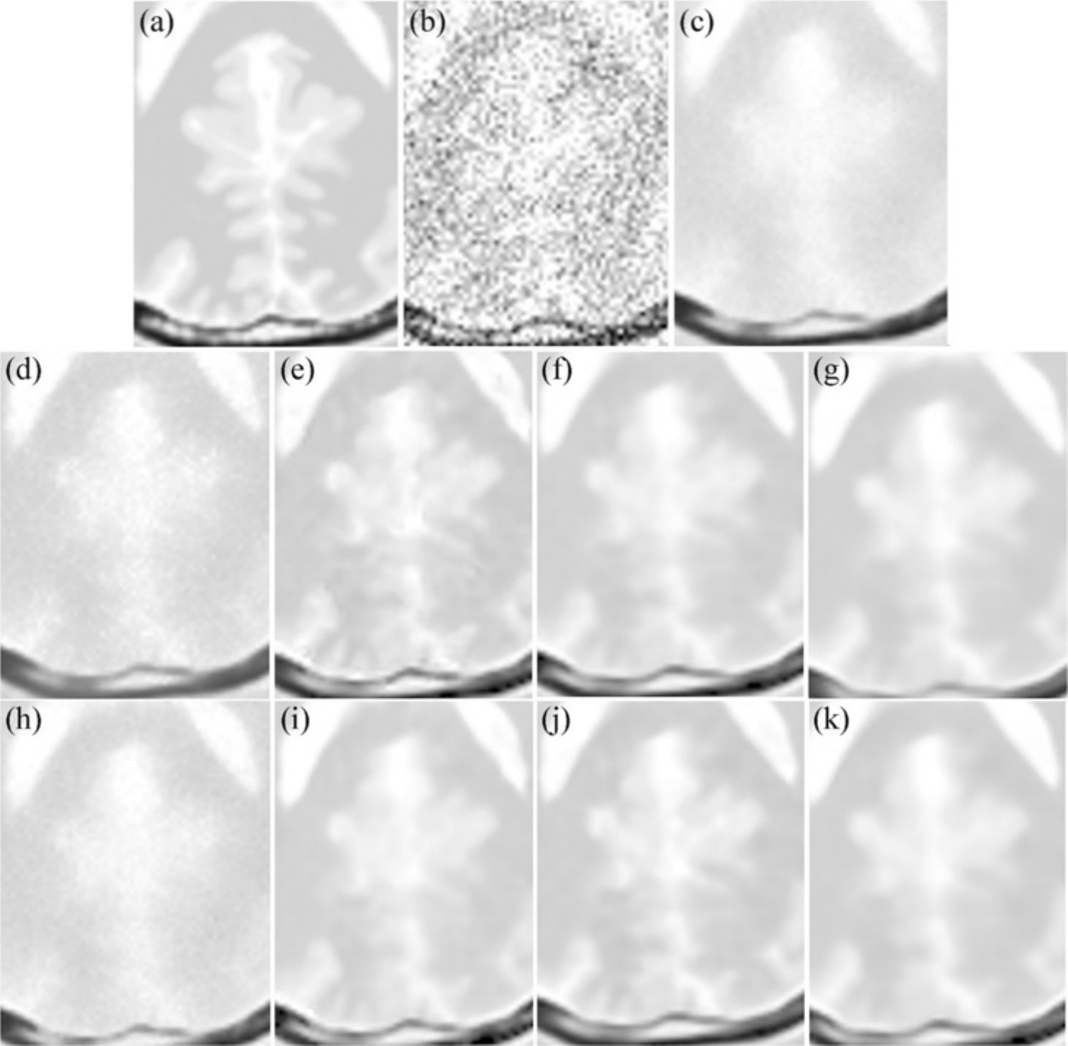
Comparison of experiment results on PD-weighted MRI. (a) original MRI, (b) noisy MRI with 17% Rician noise, (c) results of the original NLM filter, (d) results of the UNLM with squared magnitude transformation, (e) results of the PSNLM1 with median filter, (f) results of the PSNLM1 with Gaussian filter, (g) results of the PSNLM1 with anisotropic filter, (h) results of the UNLM with VST, (i) results of the PSNLM2 with median filter, (j) results of the PSNLM2 with Gaussian filter, (k) results of the PSNLM2 with anisotropic filter.
